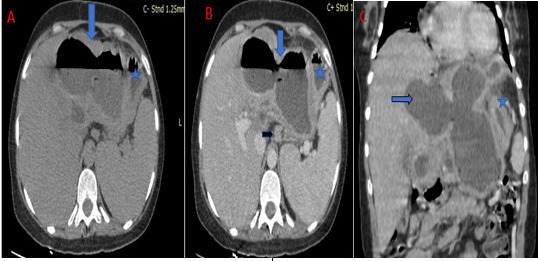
Abdominal CT scan in axial (A, B) and coronal (C)
